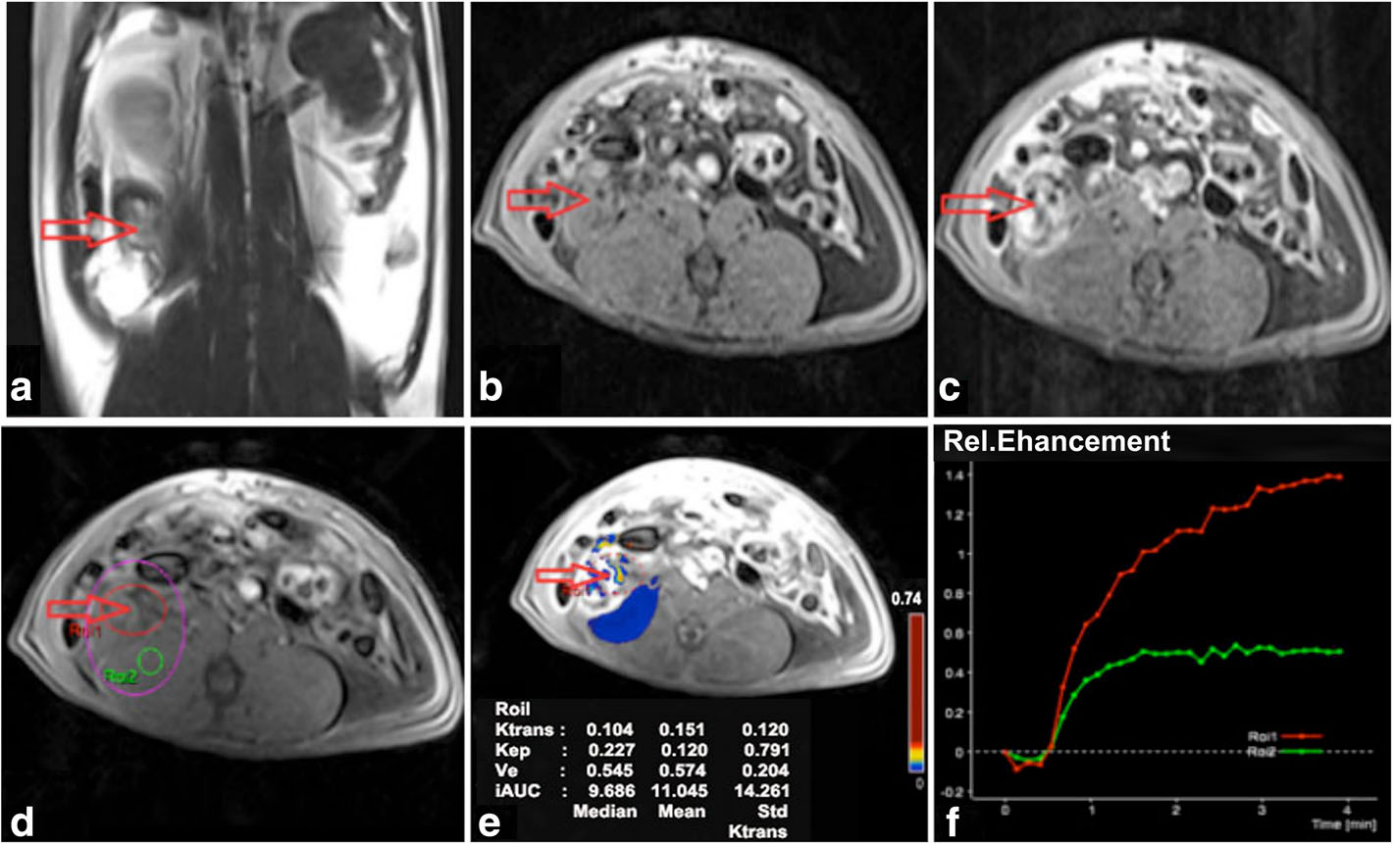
DCE-MRI imaging of EOCs with TIC II. In the right adnexal area, a multilocular cystic-solid was observed on T2WI with fat suppression (a) and T1WI (b) on the coronal position, showing obvious enhancement and solid portion on contrast-enhanced T1WI with fat suppression (c). In DCE-MRI images, ROI, including ROI1 (ovarian lesion) and ROI2 (muscle), were selected (d); pseudo-color images can be obtained by quantitatively analyzing DCE-MRI (e); the dynamic curve appeared as a moderate initial increase followed by a plateau (f). The red arrow points to the tumor focus
